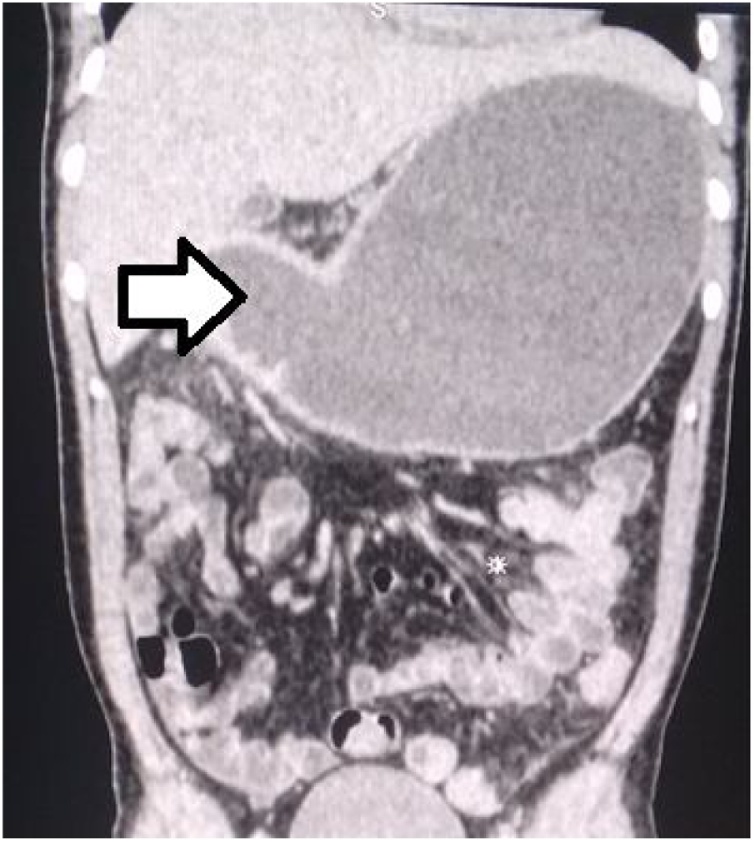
Computed tomography scan with contrast: The arrow points to the stomach that shows a severe dilatation, suggesting an associated pyloric stenosis.
